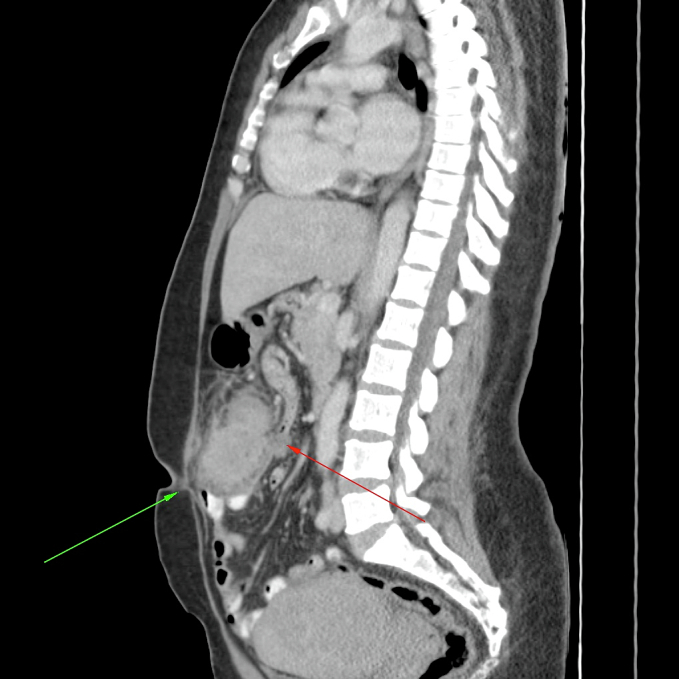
Sagittal CT view showing invasion of the mass into the peri-umbilical skin (green) and attachment to loops of small bowel posteriorly (red). (For interpretation of the references to colour in this figure legend, the reader is referred to the web version of this article.)
